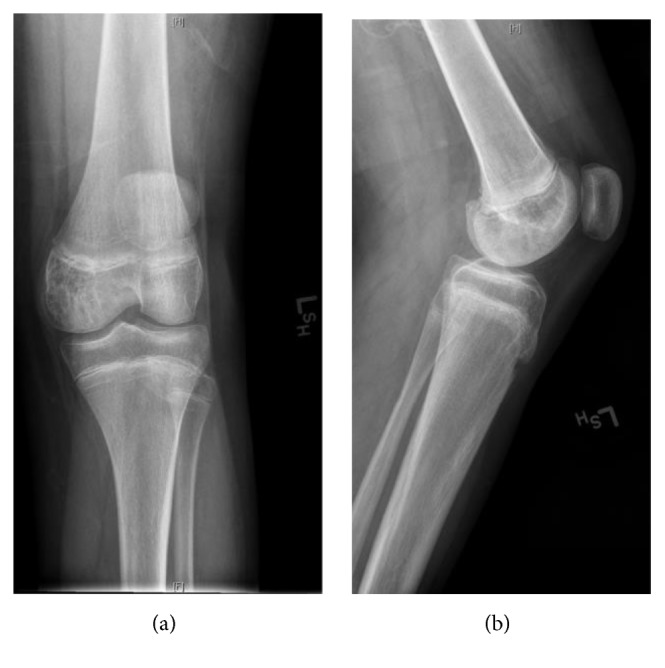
Anteroposterior (a) and lateral (b) radiographs of the left knee after chemotherapy, October 2010. Dramatic lesion response after treatment.
